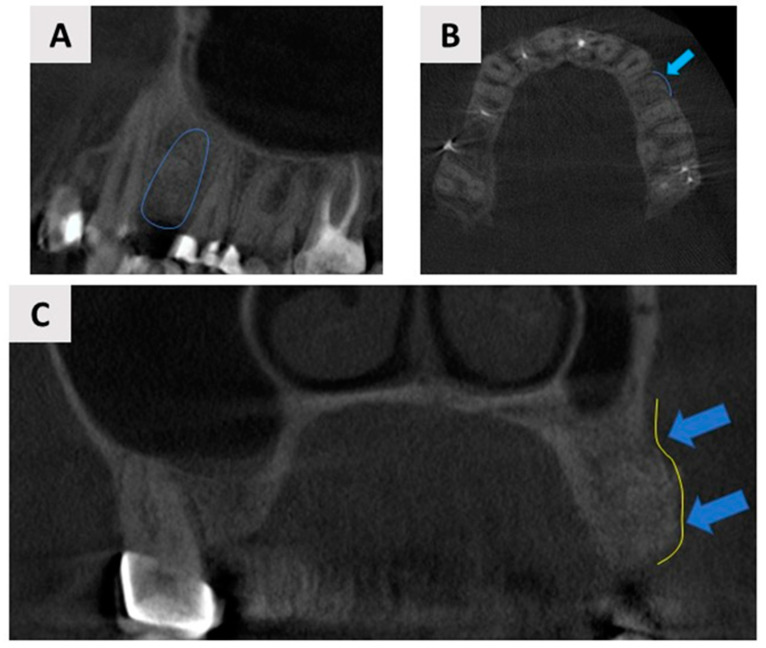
Cone-beam computed tomography (CBCT) assessment of the augmented site 6 months post-surgery. The 3D CBCT scan demonstrated satisfactory bone volume for dental implant placement. (A) Panoramic view of the augmented site, with the blue outline indicating the area of regeneration where the dental implant will be placed; (B) Axial view showing the regenerated buccal bone wall, highlighted by the blue arrows and outline; (C) Coronal plane, with blue arrows and outlines marking the newly regenerated buccal bone wall.
